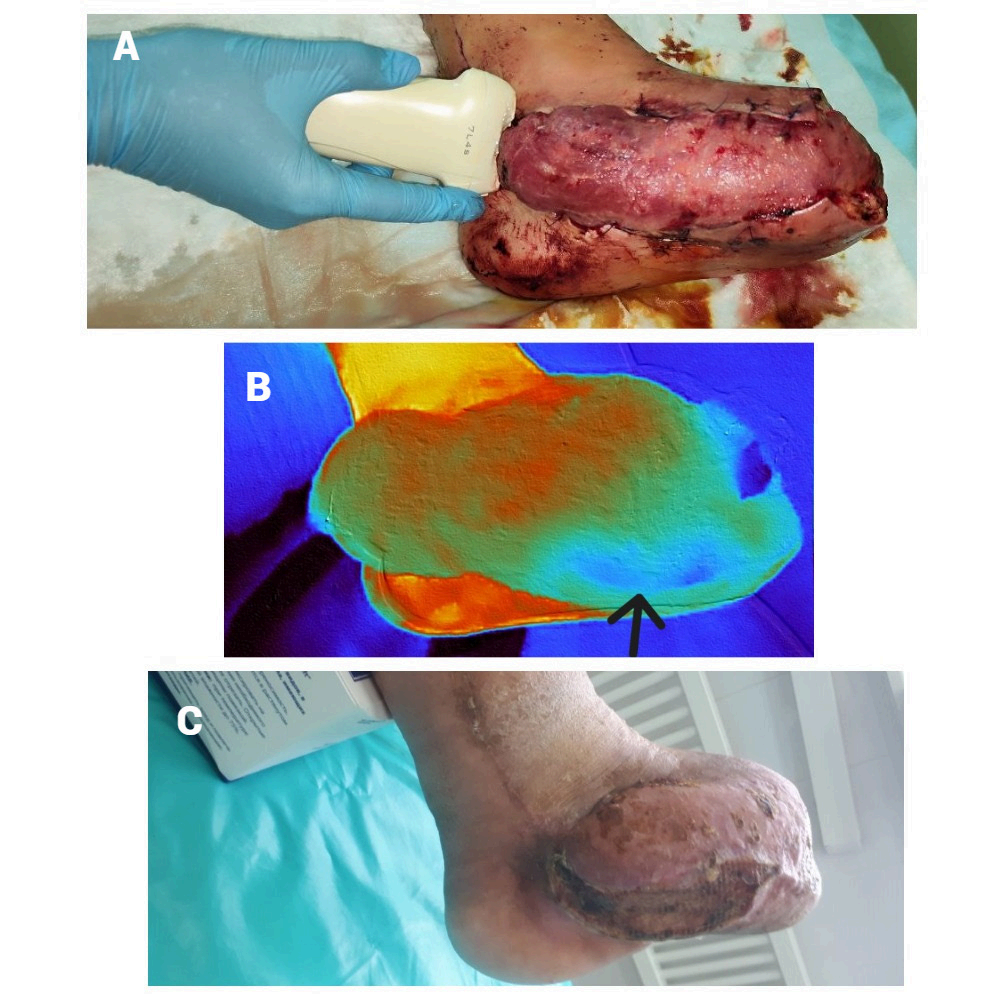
(a) Ultrasound monitoring of vascular pedicle patency; (b) thermography to assess flap viability; a hypoperfusion zone is visible, which subsequently developed partial necrosis; (c) result after 5 months.
